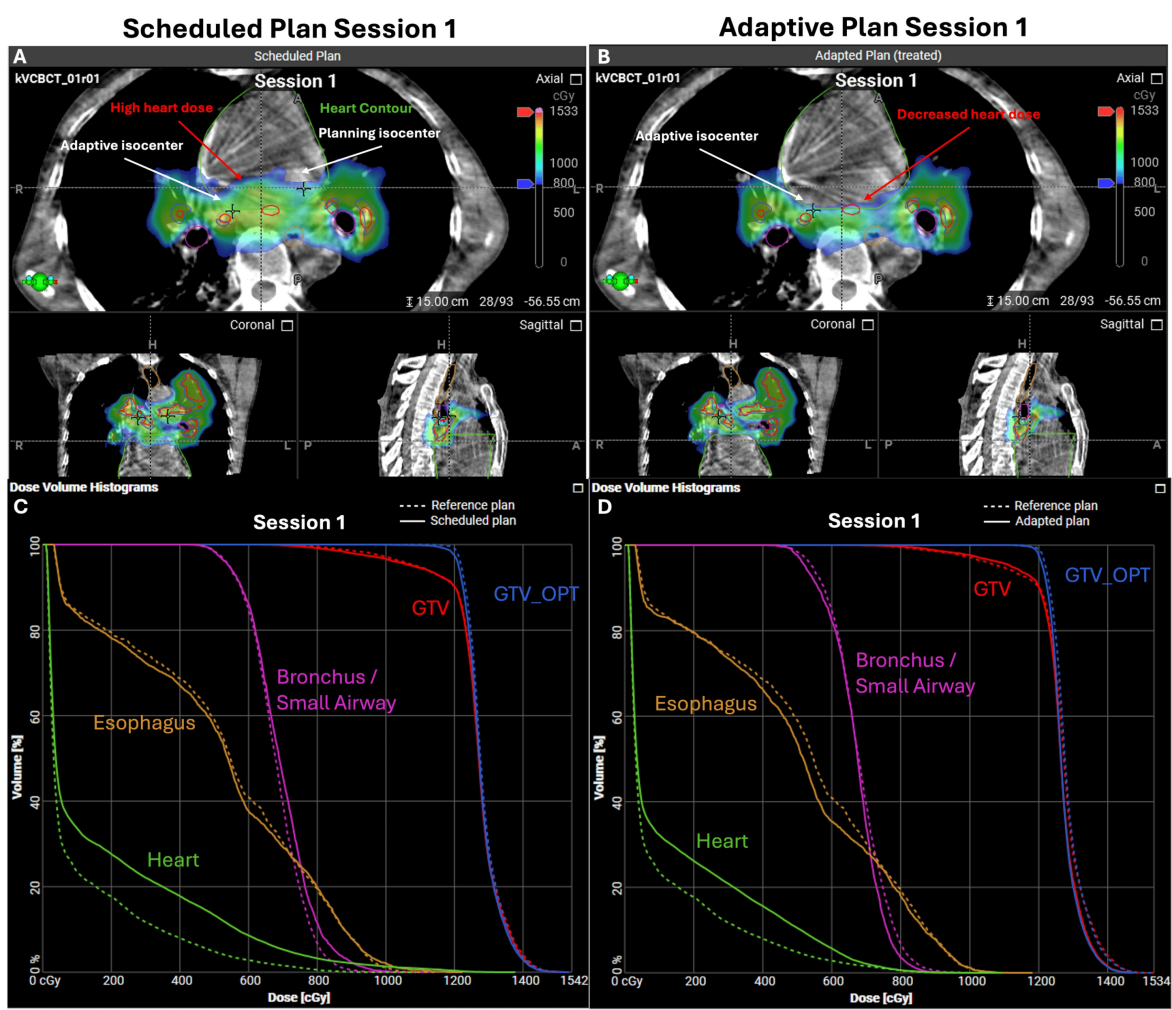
Comparison of reference, scheduled, and adaptive plans on Ethos The cone-beam CT scan and dose color wash of the (A) non-adapted scheduled plan and (B) adapted plan with a cutoff set to 800 cGy per fraction. A comparison of the dose-volume histograms of the dose to the heart (green) in the (C) non-adapted plan shows a greater heart dose (green) in the scheduled plan (solid line) to the reference plan (dashed line). The (D) adapted plan (solid line) showed a lower heart dose compared to the (C) non-adapted plan (solid line) and a smaller increase over the reference plan (dashed line). GTV: gross tumor volume; GTV_OPT: gross tumor volume optimized (PTV_OPT – 0.3 cm); PTV_OPT (planning target volume – (Bronchus/Small Airway +0.3 cm) – (Heart/Pericardium + 0.2 cm) – Trachea Large Bronchus).
